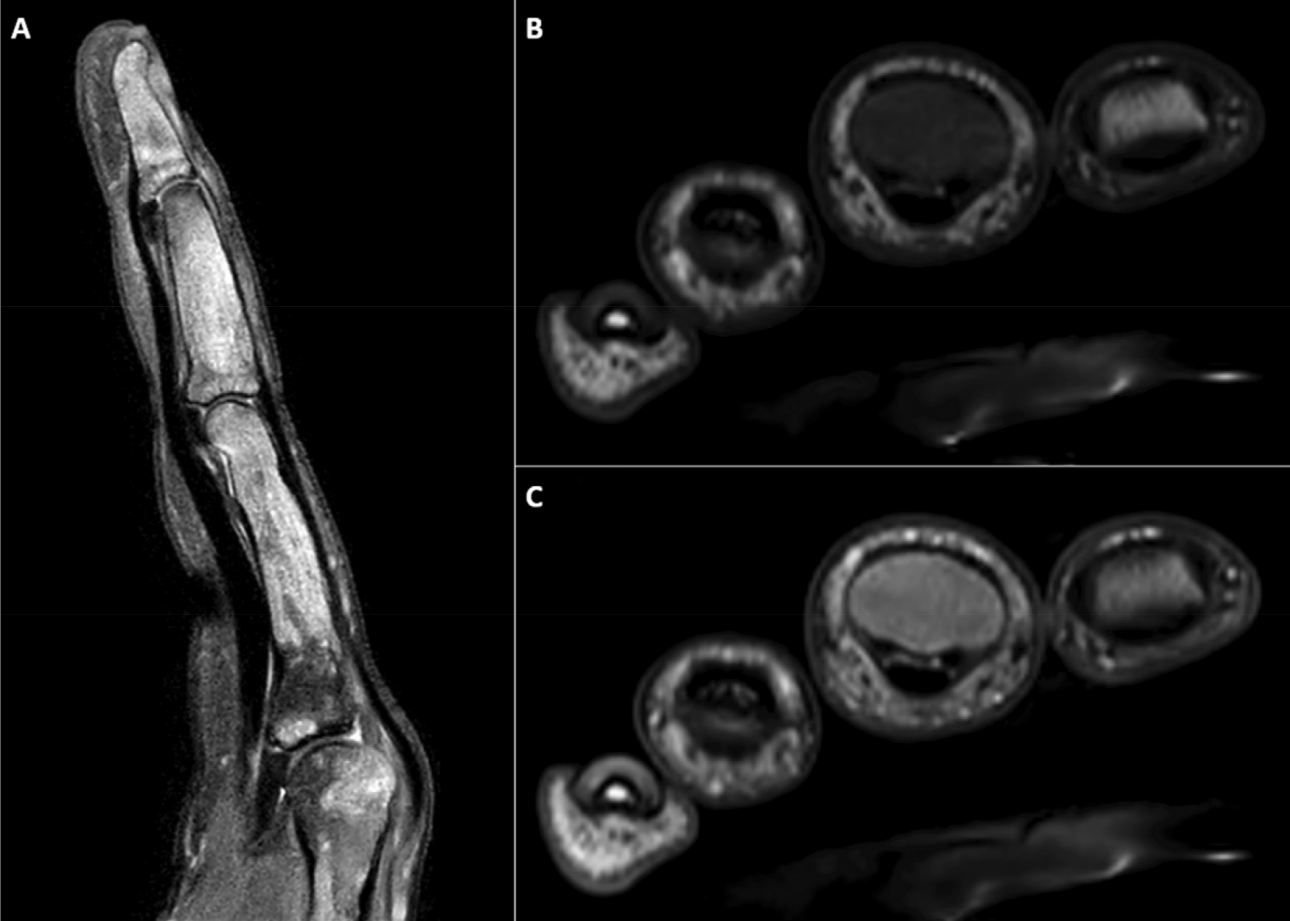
MRI of the left hand demonstrating multiple T2-hyperintense (A), T1-hypointense (B), and enhancing (C) lesions involving the phalanges and metacarpal head, leading to trabecular expansion and cortical thinning.
